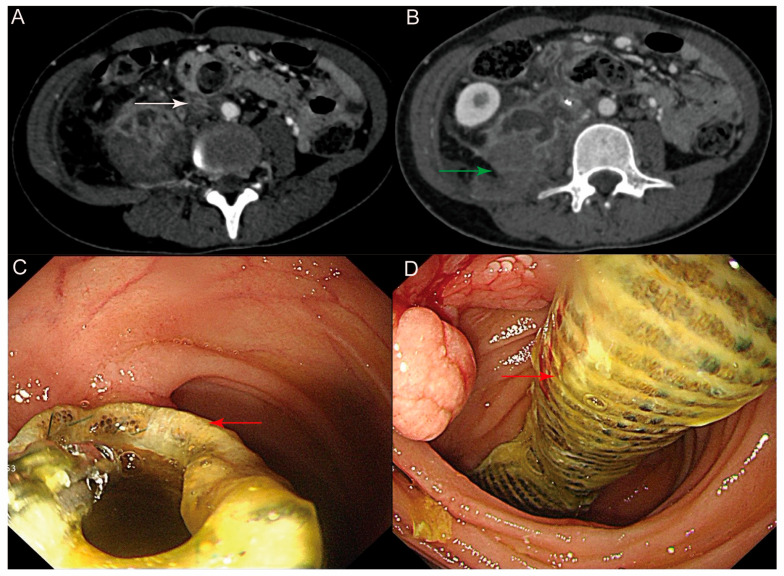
Diagnostic imaging and endoscopic findings. (A) Contrast-enhanced computed tomography showing loss of the fat plane between the inferior vena cava graft and adjacent bowel (white arrow); (B) multiloculated right psoas abscess (green arrow); (C) upper gastrointestinal endoscopy showing the proximal graft segment protruding into the descending duodenum (red arrow); (D) prosthetic graft material exposed within the duodenal lumen, confirming graft enteric fistulization (red arrow).
